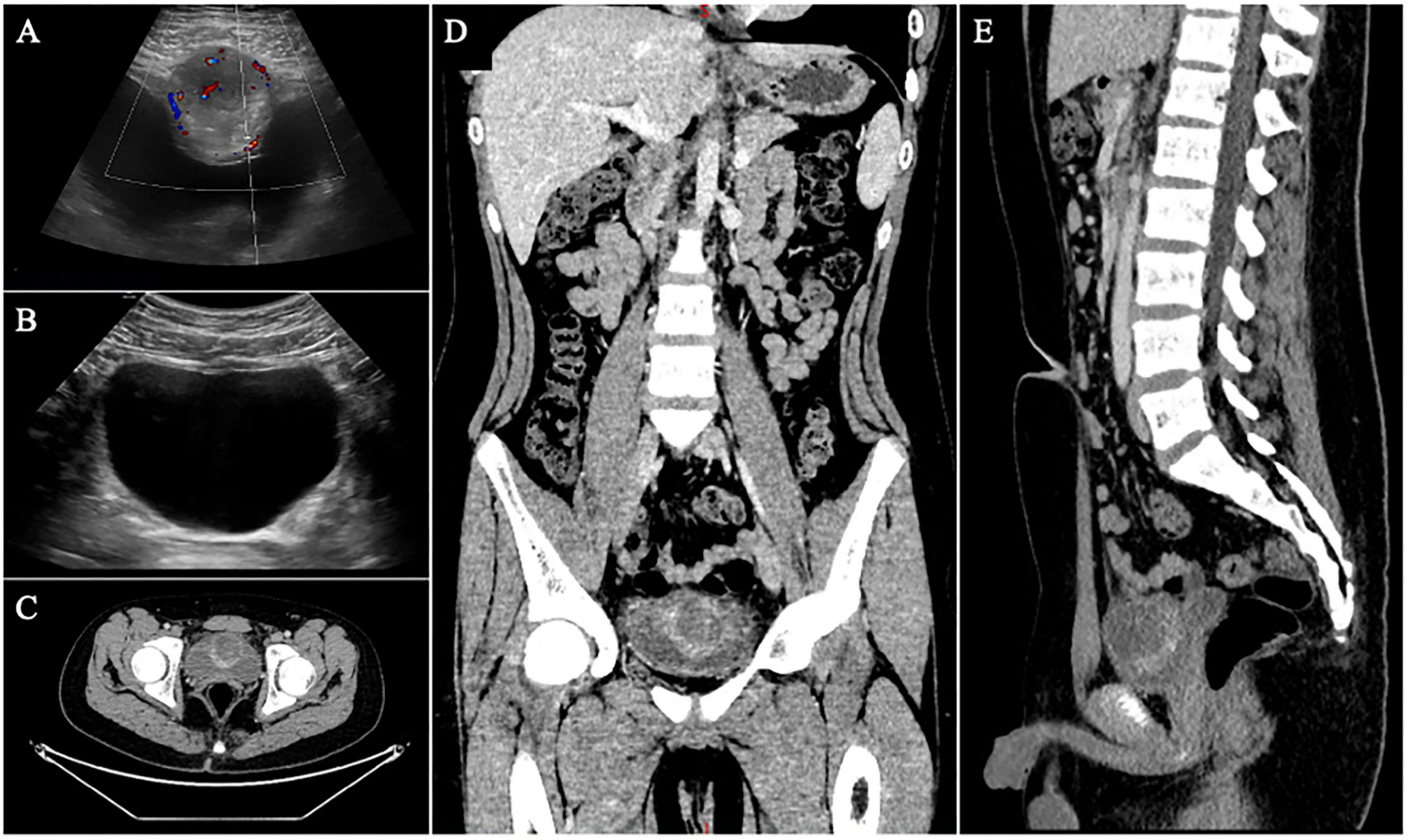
Imaging findings. (A) Preoperative ultrasound demonstrated a cystic lesion protruding into the bladder lumen from the anterior wall. The bladder mucosa is irregular, with increased echogenicity in the surrounding soft tissue and abundant blood flow signals in the superior wall. (B) At 6-month postoperative follow-up, ultrasound showed focal blurring of the anterior bladder wall with no evidence of abnormal echoic lesions. (C–E). Preoperative contrast-enhanced CT showed an ovoid mass in the anterosuperior bladder wall with heterogeneous peripheral rim enhancement.
